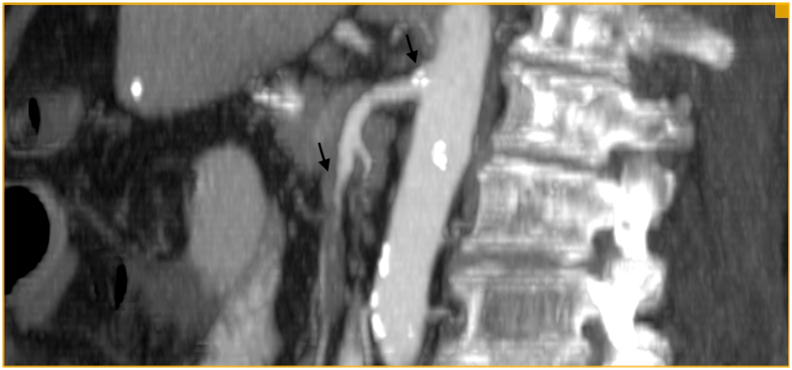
Preoperative CT-angiography showing superior mesenteric artery (SMA) origin stenosis and occlusion after 2 cm from the origin.
